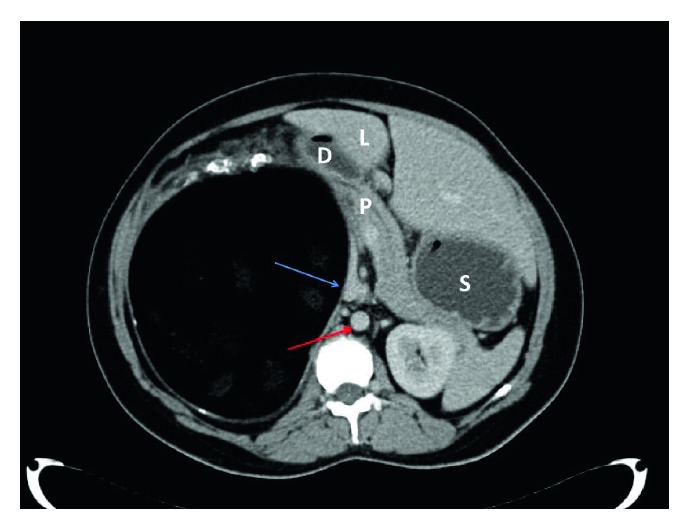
CT scan of the abdomen (axial view) demonstrating the complex cystic mass that appears to arise from the retroperitoneum and displaces the left liver (L), pancreatic head (P), duodenum (D), and inferior vena cava (blue arrow) anteromedially. For orientation, the stomach (S) and aorta (red arrow) are labeled.
